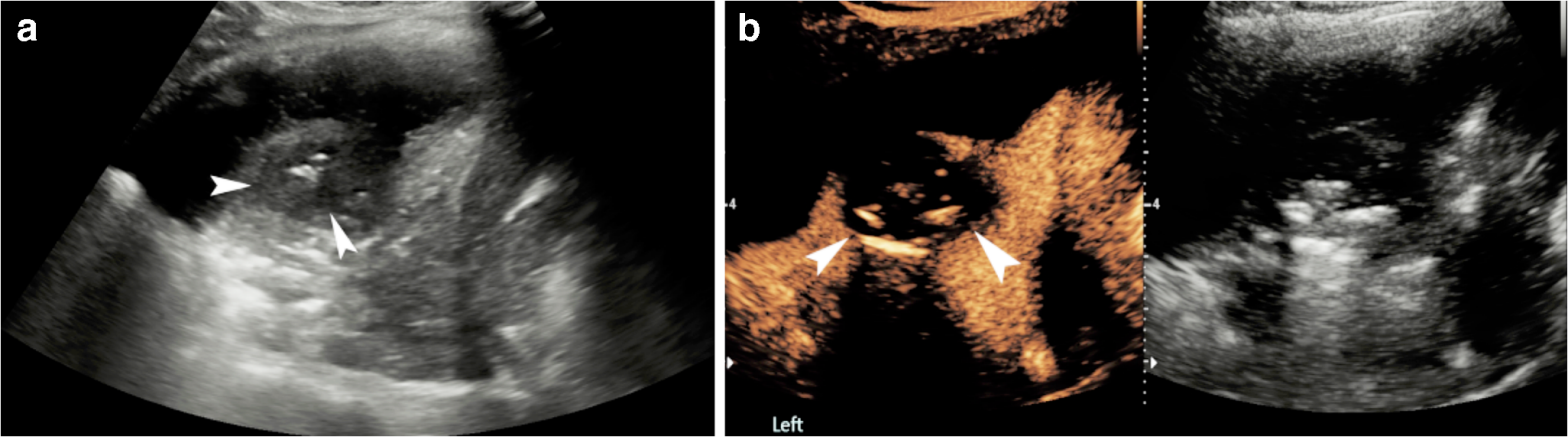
Worsening symptoms in a 2-year old girl hospitalized with community-acquired pneumonia. a Gray-scale US of the left lung in coronal plane shows a heterogeneous appearance of the visualized lung parenchyma in the left lung base, with a round, relatively hypoechoic region (*arrowheads*) centrally within the consolidated lung, containing some echogenic foci, attributable to air bronchogram. Adjacent to the consolidation there is a large volume of pleural effusion, with no significant loculations or debris. b Contrast-enhanced ultrasound (CEUS) dual-screen mode with simultaneous display of contrast mode (*left*) and gray-scale mode (*right*), following intravenous administration of US contrast agent. Coronal view of the left lung shows enhancement of the consolidated lung, but the central area shows absence of enhancement (*arrowheads*) with no definite wall or rim enhancement, suggestive of necrotizing pneumonia
